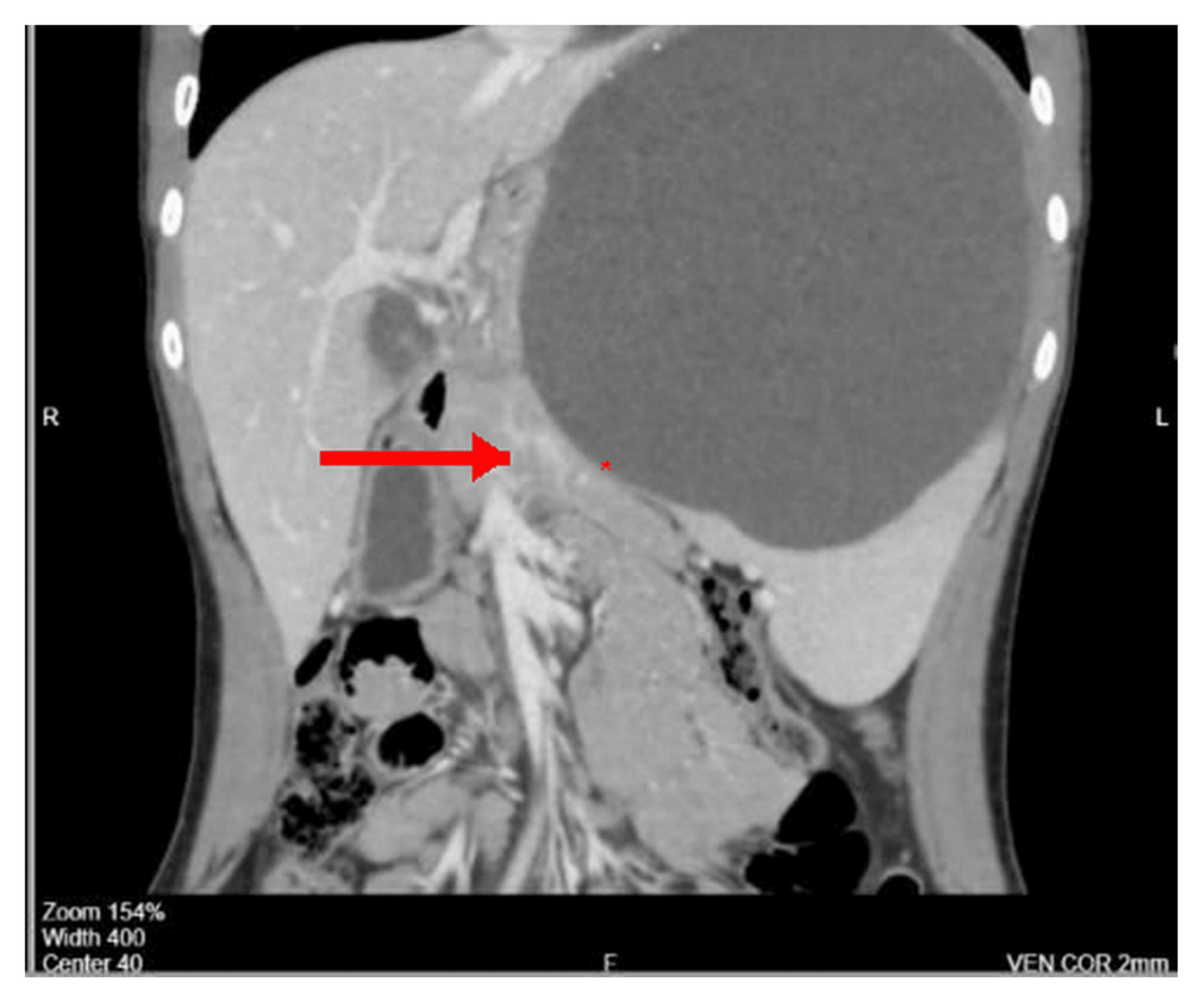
Coronal CT scan showing a large thin-walled splenic cyst occupying the upper pole with peripheral calcifications. Axial contrast-enhanced CT scan of the abdomen showing a giant splenic cyst occupying the upper pole of the spleen, causing displacement of adjacent structures including the stomach and left kidney (asterisk marks cyst cavity).
